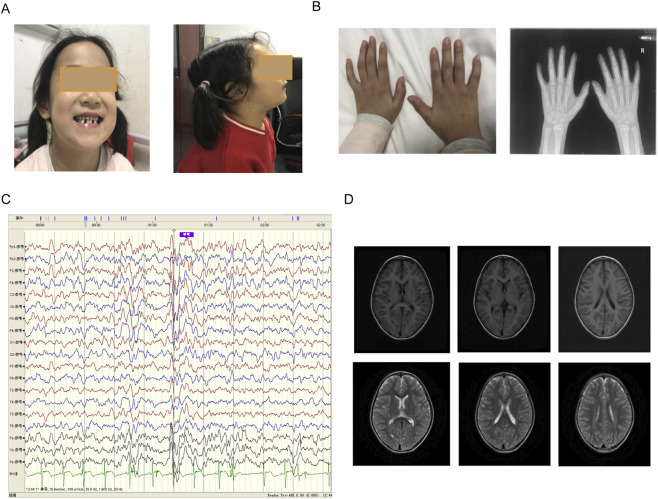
Clinical presentation of the patient. (A) Frontal and lateral views of the patient. (B) Hands and fingers of the patient. (C) EEG at the age of 5 years. (D) Brain MRI at the age of 5 years.
